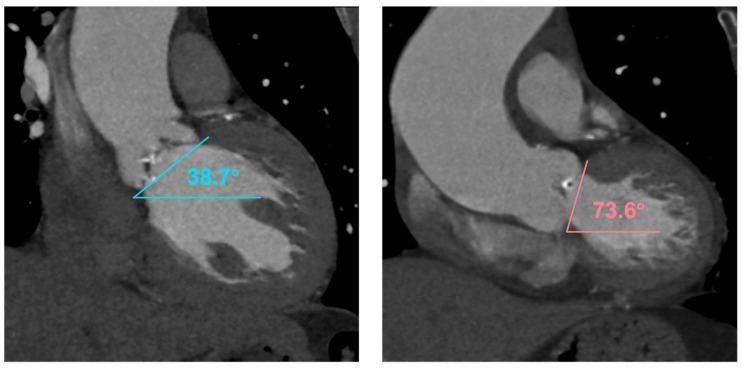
Measurement of annulus angulation on CT. Annulus angulation was measured as the angle between the horizontal plane and the annulus plane on coronal projection of reconstructed computed tomographic images. A representative image of non-horizontal and horizontal aorta was presented.
